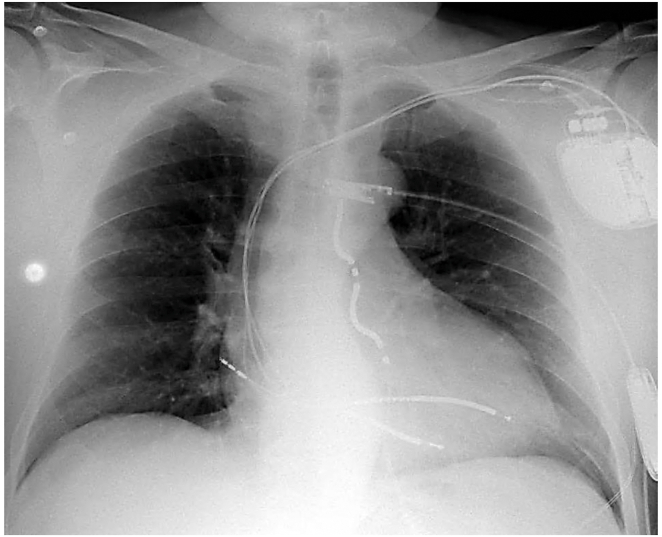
Postoperative chest radiograph showing a dual-chamber endocardial implantable cardioverter-defibrillator, an abandoned right ventricular lead, and an extravascular implantable cardioverter-defibrillator system.
